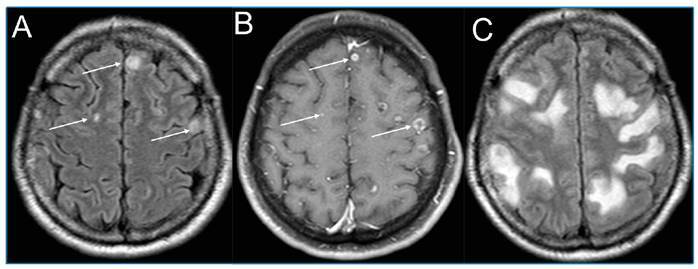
(A) MRI shows small peripheral lesions on FLAIR. (B) MRI shows small peripheral lesions with annular contrast enhancement on T1WI post-contrast. (C) The new MRI shows increased expansive mass effect and vasogenic edema on FLAIR. FLAIR: fluid attenuated inversion recovery. T1WI: T1-weighted image.
